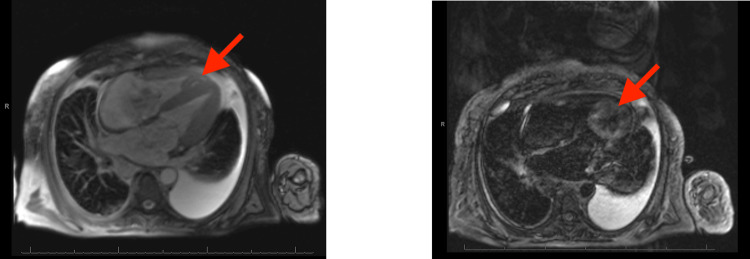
Cardiac MRI T2 weighted images in a short axis plane, resting images were completed with intravenous (IV) multihance contrast. A few focal areas of enhancement were located within the septum (shown in arrow).
